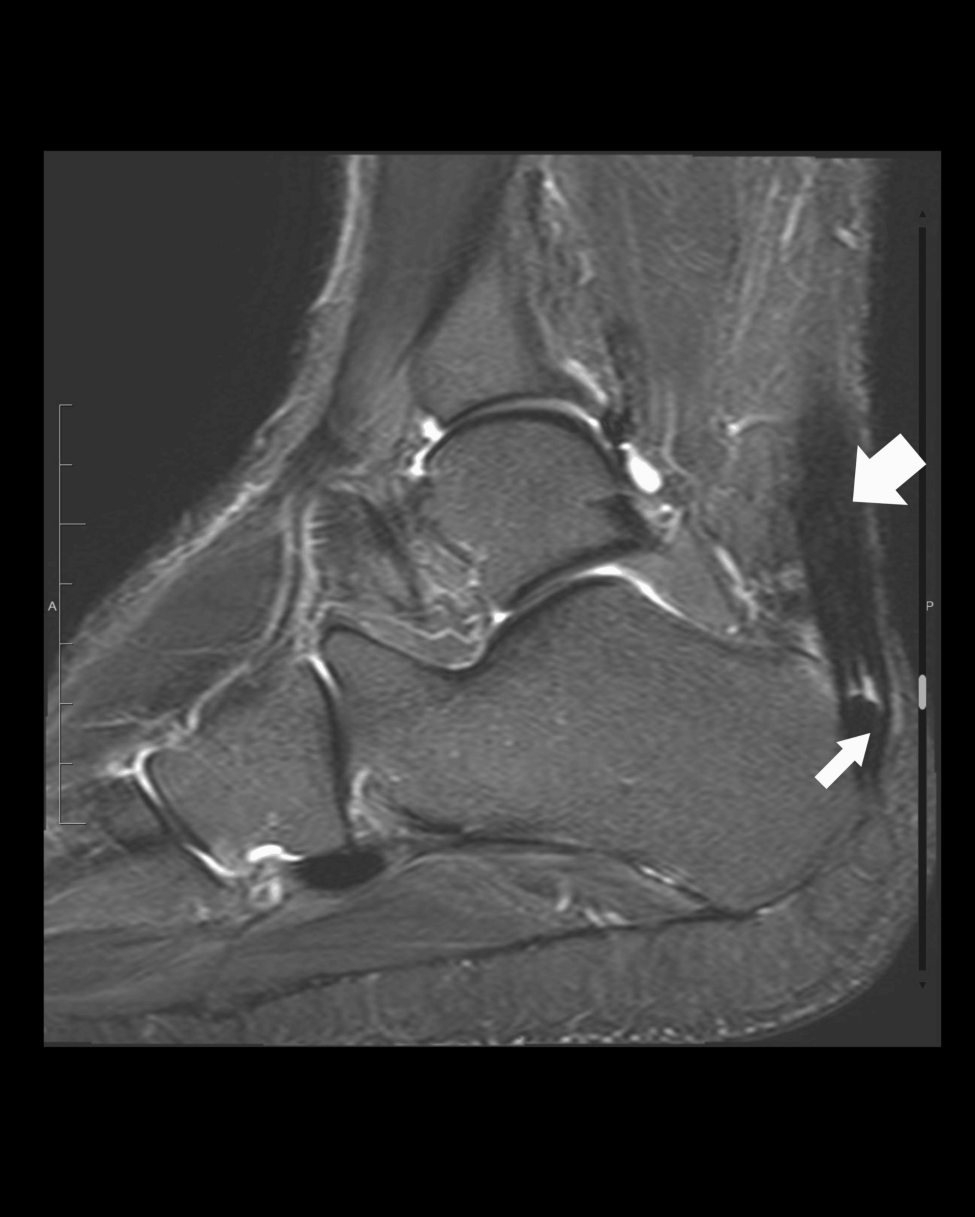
Radiological findings at patient presentation. Left ankle MRI sagittal STIR image showing chronic tendinopathy (bold arrow) with a partial tear of the Achilles tendon near the calcaneal insertion (thin arrow).
